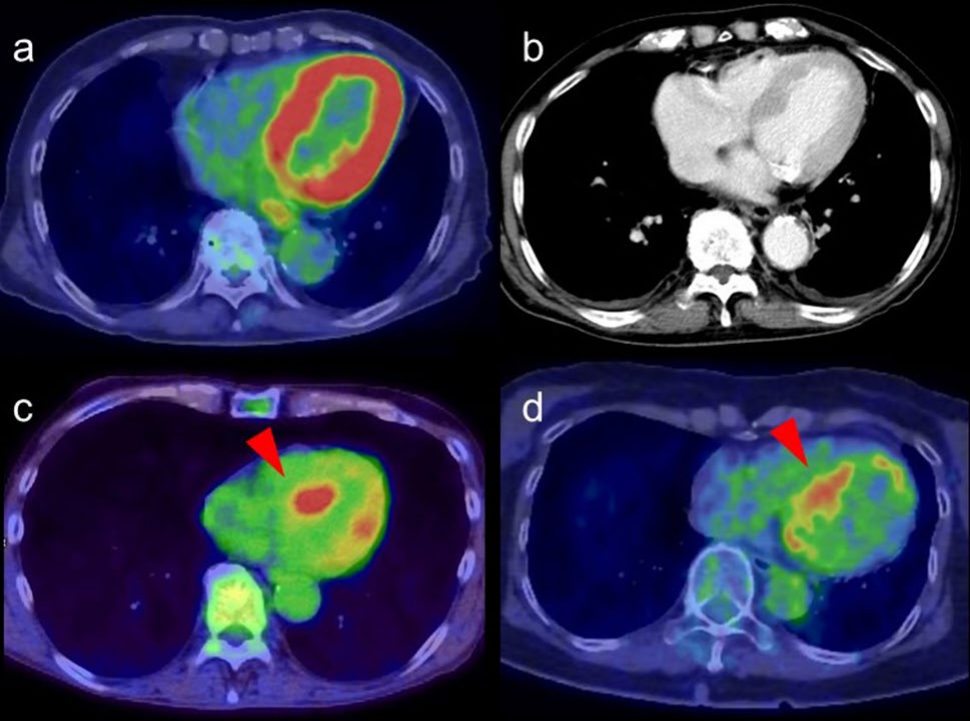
FDG uptake in left ventricular hypertrophy. Intense FDG uptake was confirmed along with the left ventricular wall (a). Contrast enhanced CT suggested thickening of left ventricular wall suggesting left ventricular hypertrophy (b). Myocardial FDG uptake features are shown with hypertrophic obstructive cardiomyopathy (c) and with hypertrophic nonobstructive cardiomyopathy (d) (red arrowheads)
